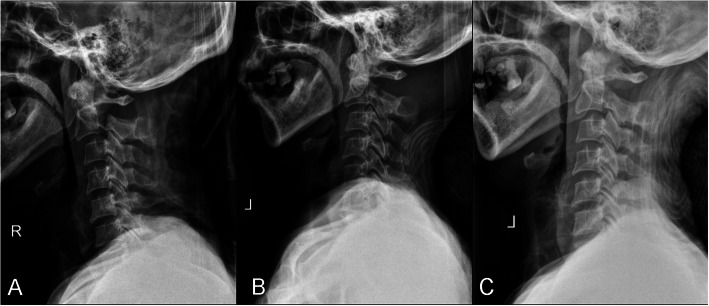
a Lateral radiograph, indicating type II Hangman’s fracture. b The patient was hospitalized with skull traction, and the lateral radiograph showed the partial reduction after skull traction for 3 days. c Lateral radiograph after 5-day skull traction, manifesting complete reduction which represented the fracture end separation was ≤ 2 mm with no obvious angle
